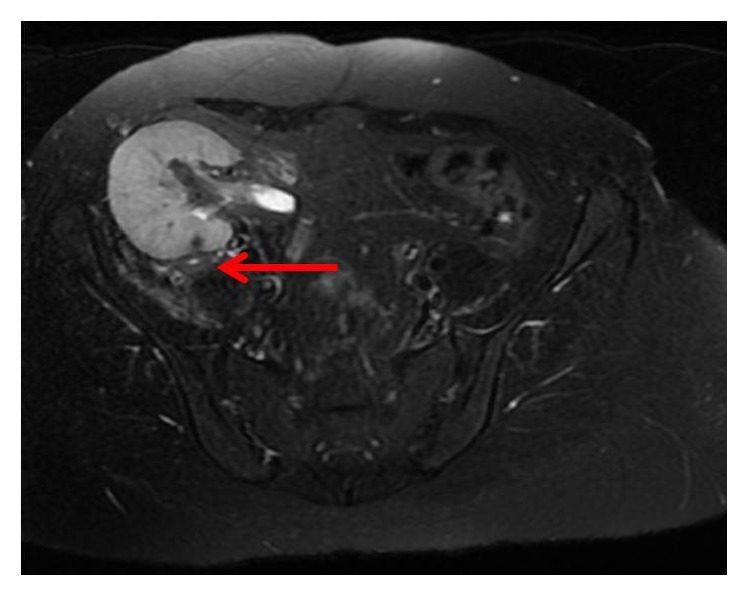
Contrast T2-weighted magnetic resonance imaging. After the patient was given antibiotics, right psoas abscess ameliorated within 2 months (red arrow).
